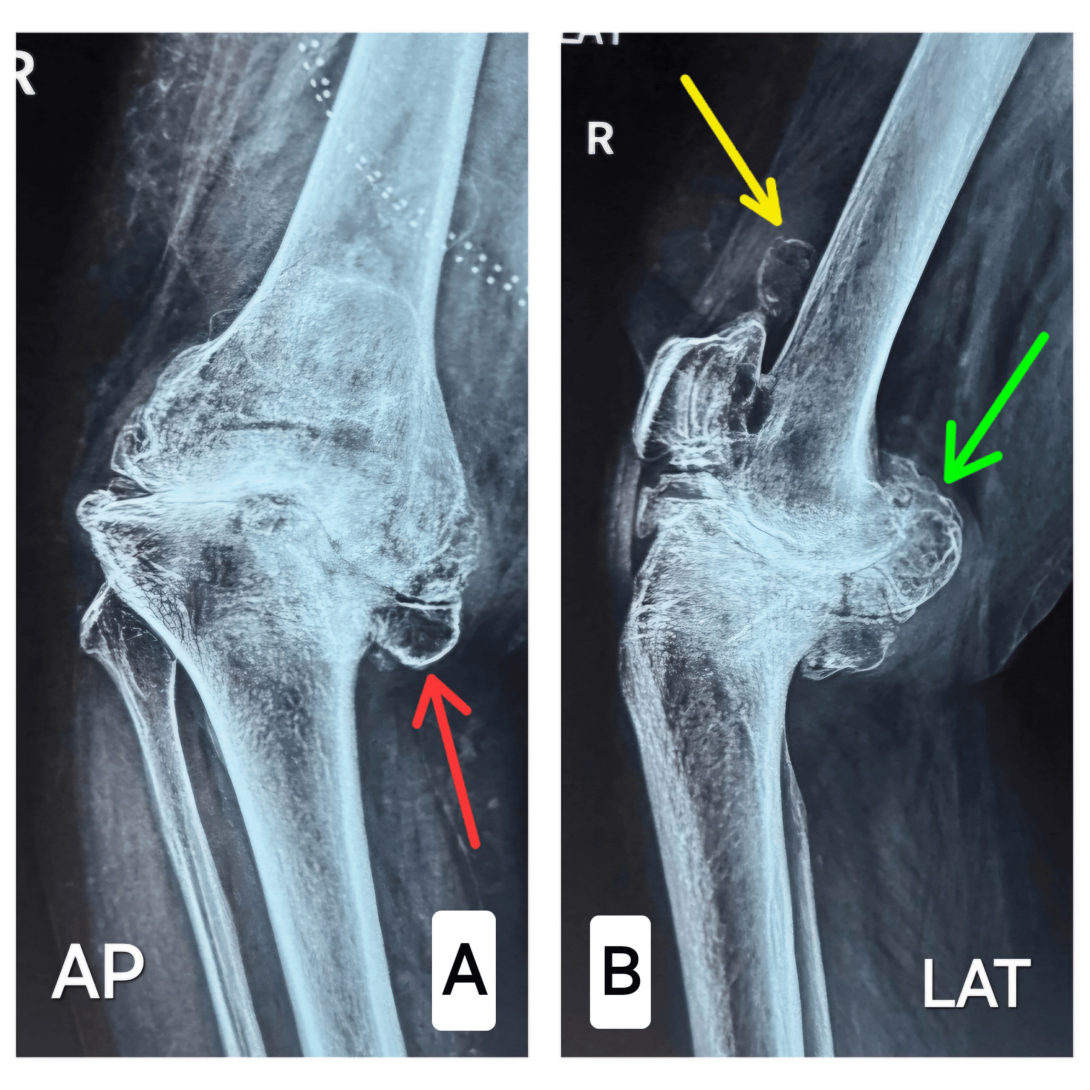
X-ray of right knee showing anteroposterior view and lateral views. A- Red arrow showing proximal tibial bone loss; B- Green arrow showing femoral osteophytes and yellow arrow showing loose body.
